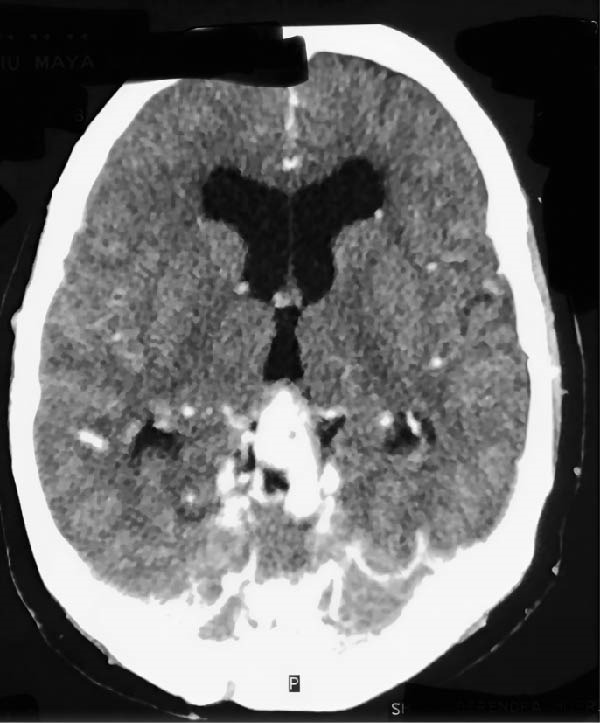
Contrast‐enhanced CT—axial. Postoperative contrast‐enhanced CT performed 2 days after surgery demonstrating reduction in nidus size.
